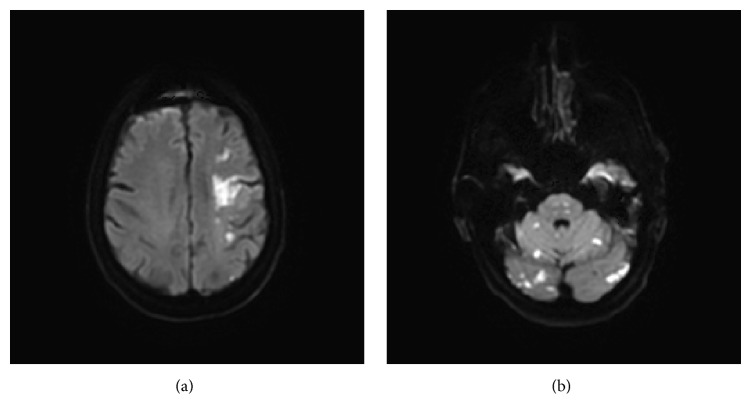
(a) An MRI of the brain revealing areas of restricted diffusion in the left carotid artery distribution. (b) MRI of the brain showing new infarcts in the superior cerebellum and occipital lobe, within the PTA territory.
